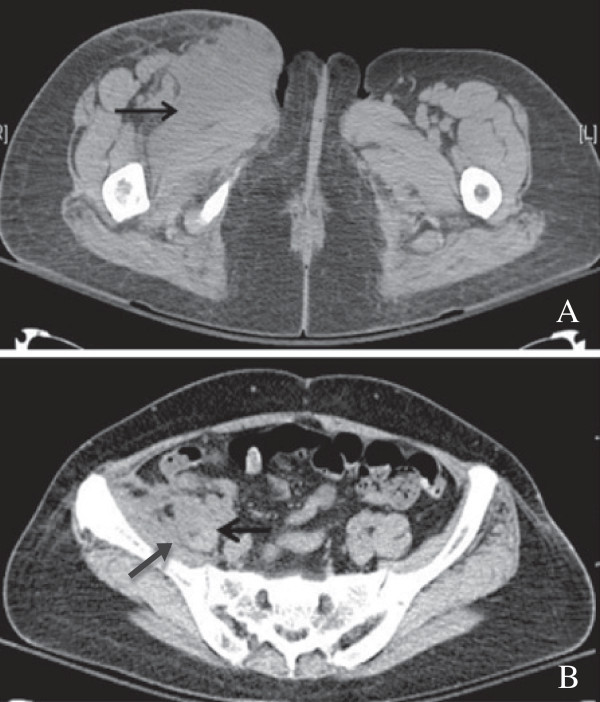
Computed tomography of the pelvis showed (A) Amorphous fluid collection with air bubbles involved proximal to the right iliopsoas muscle and distal to the adductor muscle of the right groin, (B) The right iliopsoas muscle appeared to be enlarged with an irregular margin and had some stranding adjacent to the colon.
